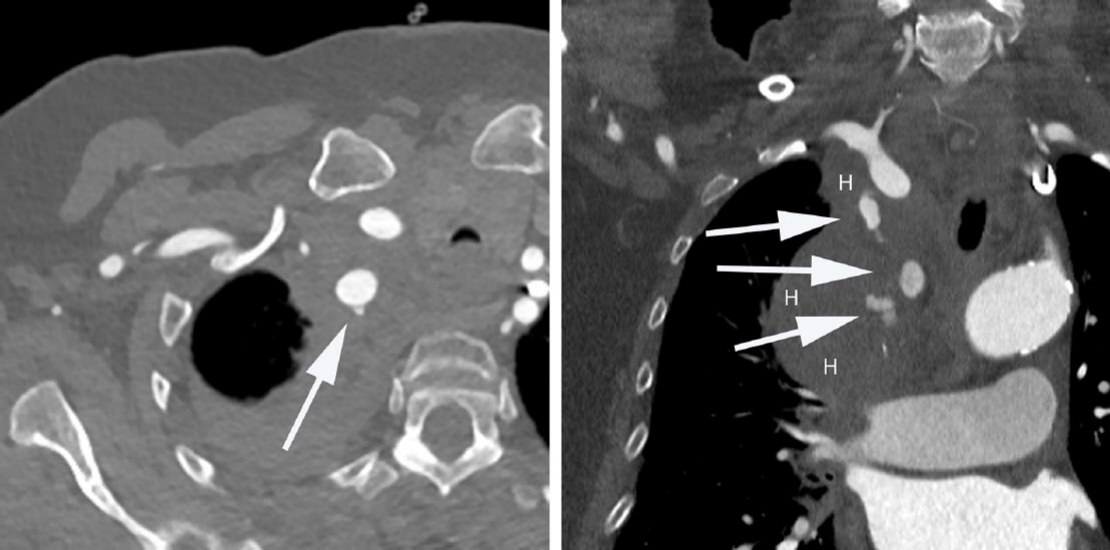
Computed tomography showing perforation of the right subclavian artery (arrows) with a mediastinal hematoma with sagittal and coronal views (H, hematoma).
